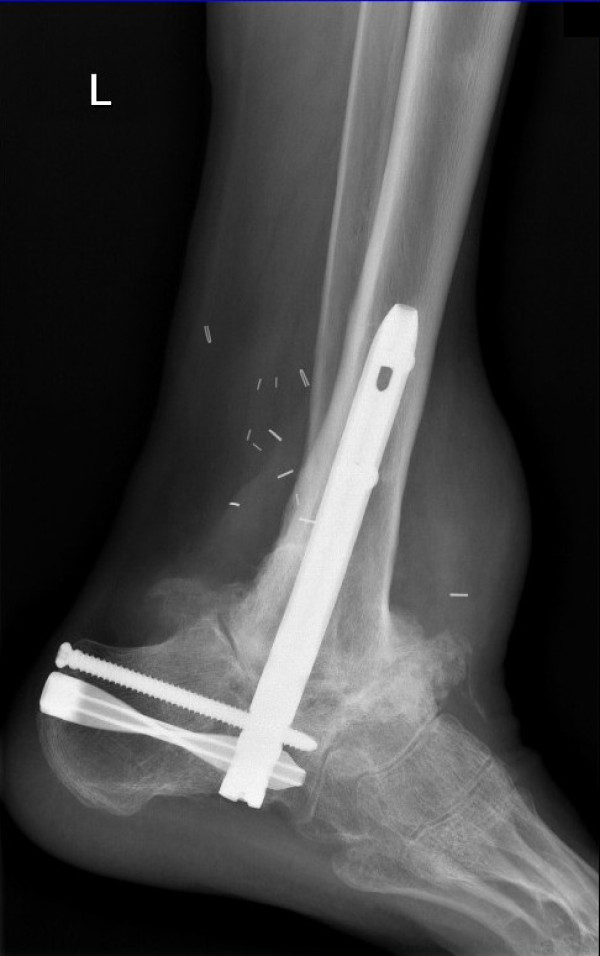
Radiograph at 21-year follow-up. The radiograph was taken six months after fixation with hindfoot arthrodesis nail and grafting.
